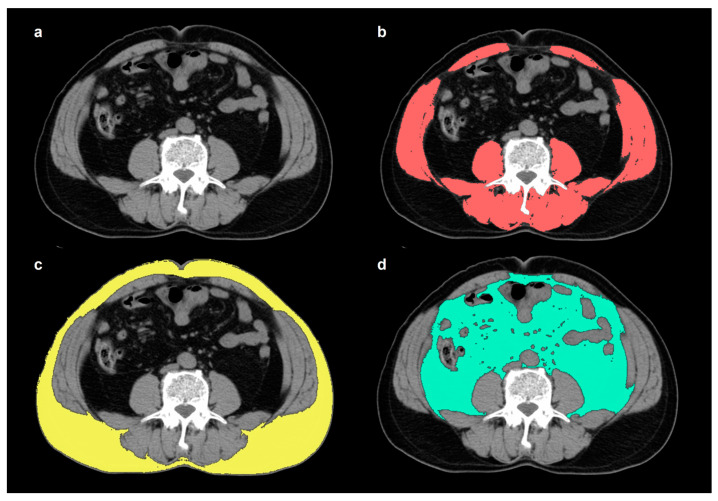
An example of the method used to calculate total skeletal muscle, SAT, and VAT areas from non-contrast-enhanced abdominopelvic CT scan images. On a transaxial CT image at the level of the L3 spine (a), the total skeletal muscle (b) area was automatically delineated using a CT-attenuation range from −29 to 150 HU (scarlet color in (b)). A CT-attenuation threshold of −190 to −30 HU was used for both SAT and VAT. SAT (c) was defined as extra-peritoneal adipose tissue between the skin and muscle (yellow color in (c)), while VAT (d) was defined as intra-abdominal adipose tissue (light green color in (d)).
